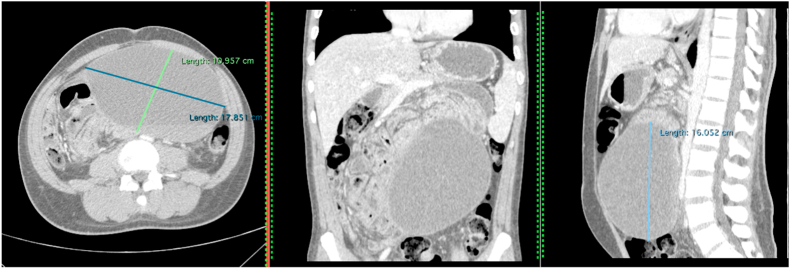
Abdomen Contrast-CT demonstrating a well-defined large cystic lesion causing compression of intestinal loops with close relation with the Treitz ligament and middle colic vessels, measuring 17, 8 × 16 × 10, 9 cm.
